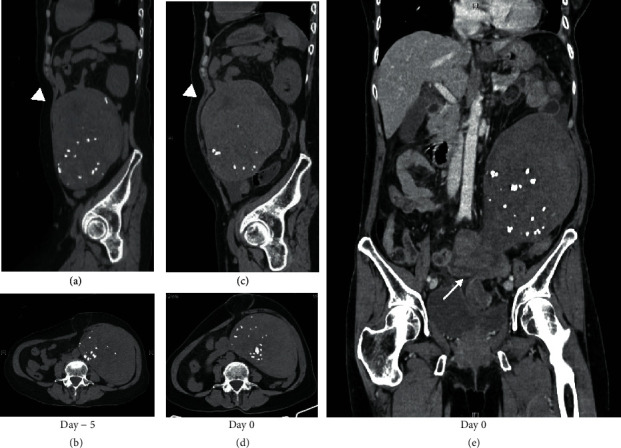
Computed tomography (CT) images of the uterus and leiomyoma. Comparison of sagittal (a, c) and axial (b, d) views of the plain abdominal CT images. Compared to the CT scan taken 5 days before the surgery (a, b), the leiomyoma had slightly enlarged in size and anteriorly deviated (white arrowheads) on the day of emergency surgery (c, d). The enhanced CT image (e) showed a twisted cervix at the level of the uterine body and cervical junction (white arrow).
